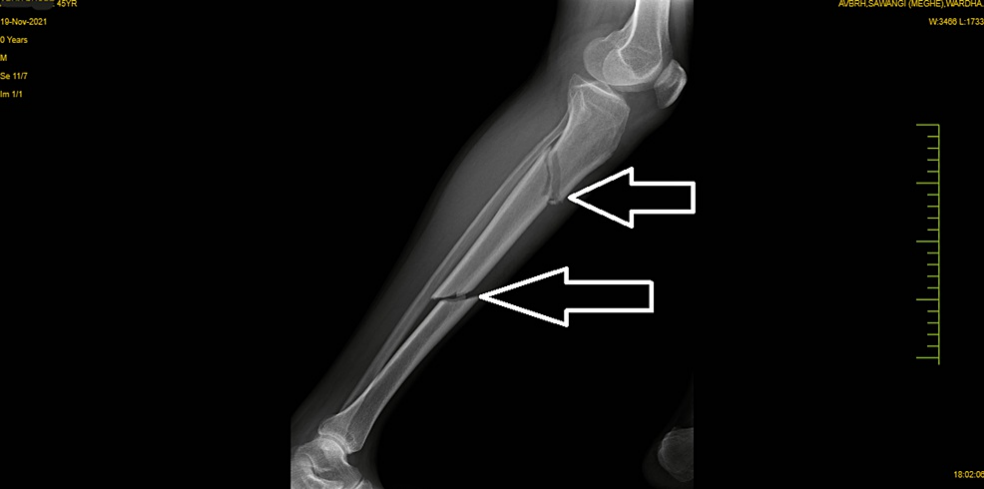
X-ray tibia (lateral view) shows a tibial shaft fracture.
